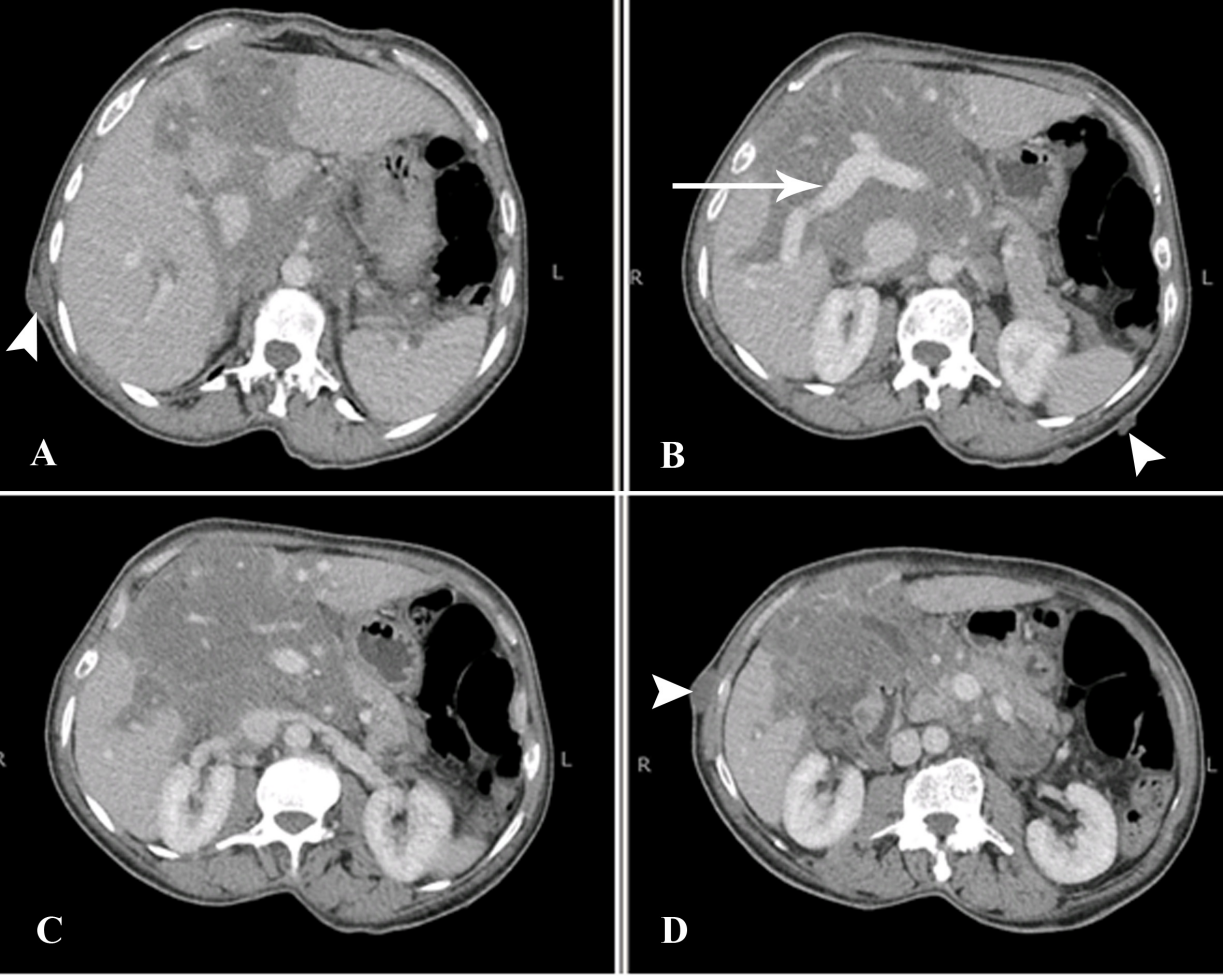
CT Abdomen Axial Sections Multilobulated infiltrative, low-attenuating, minimally enhancing mass at the porta hepatis with intrahepatic and periportal distribution, extending along the retroperitoneum, and encasing the major vessels. The portal vein is normally enhancing (arrow). Multiple cutaneous nodules are also seen, consistent with neurofibromas (arrowheads). CT - computed tomography.
